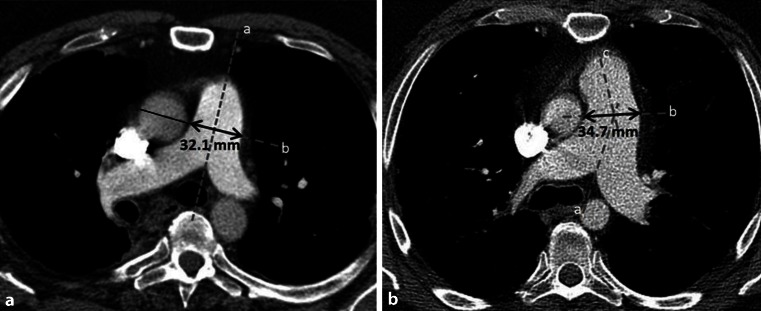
Measurement of the pulmonary artery on chest CT. a Measurement of the main pulmonary artery, at the level of the bifurcation along the line that originates from the centre of the adjacent ascending aorta, perpendicular to the axis of main pulmonary artery; b If the trunk is too curved, measurements can be made using the other method [32]
